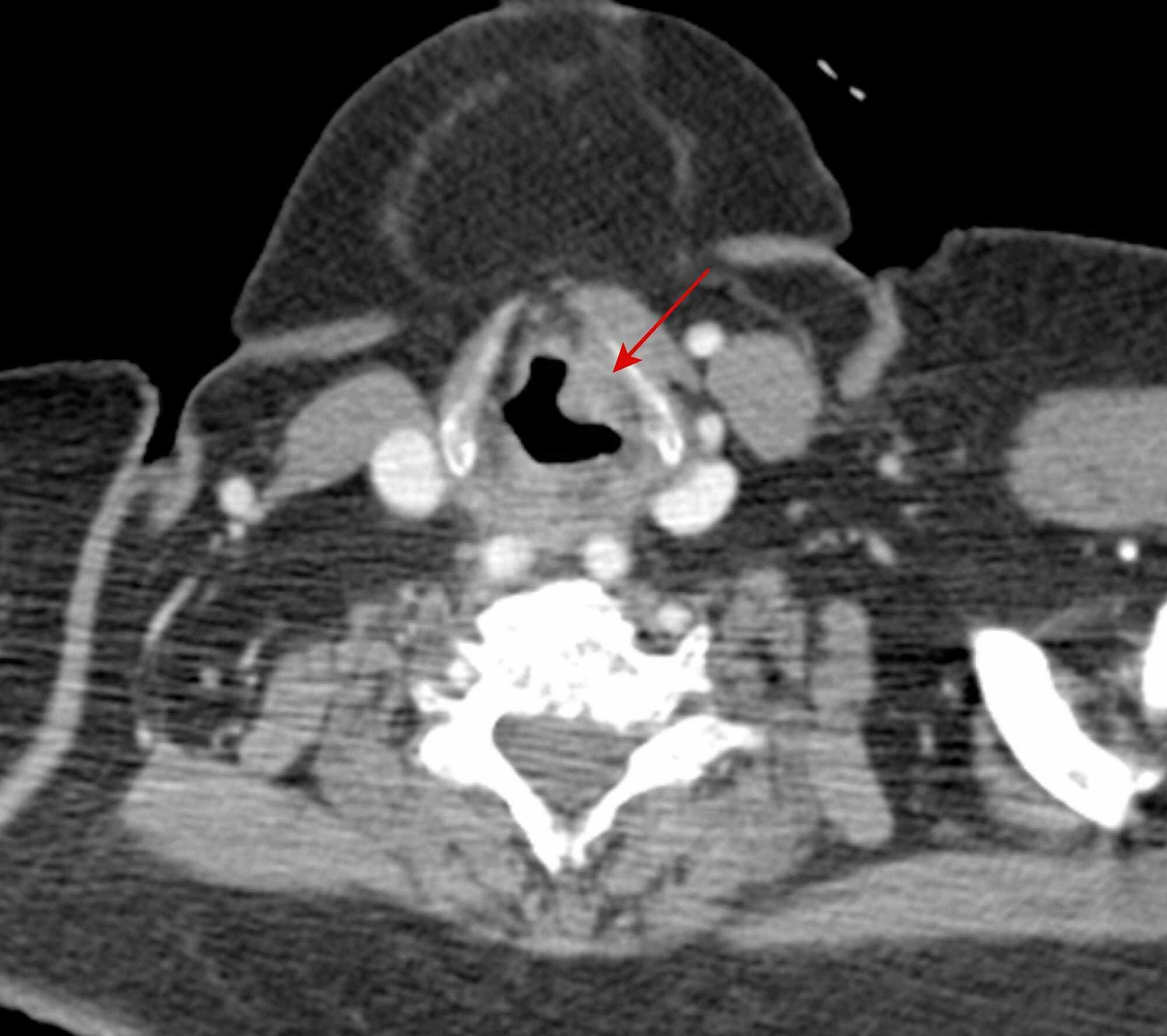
A CT scan with contrast (axial plane) conducted six months prior to surgery The mass is highlighted by the red arrow in the left supraglottic region.
